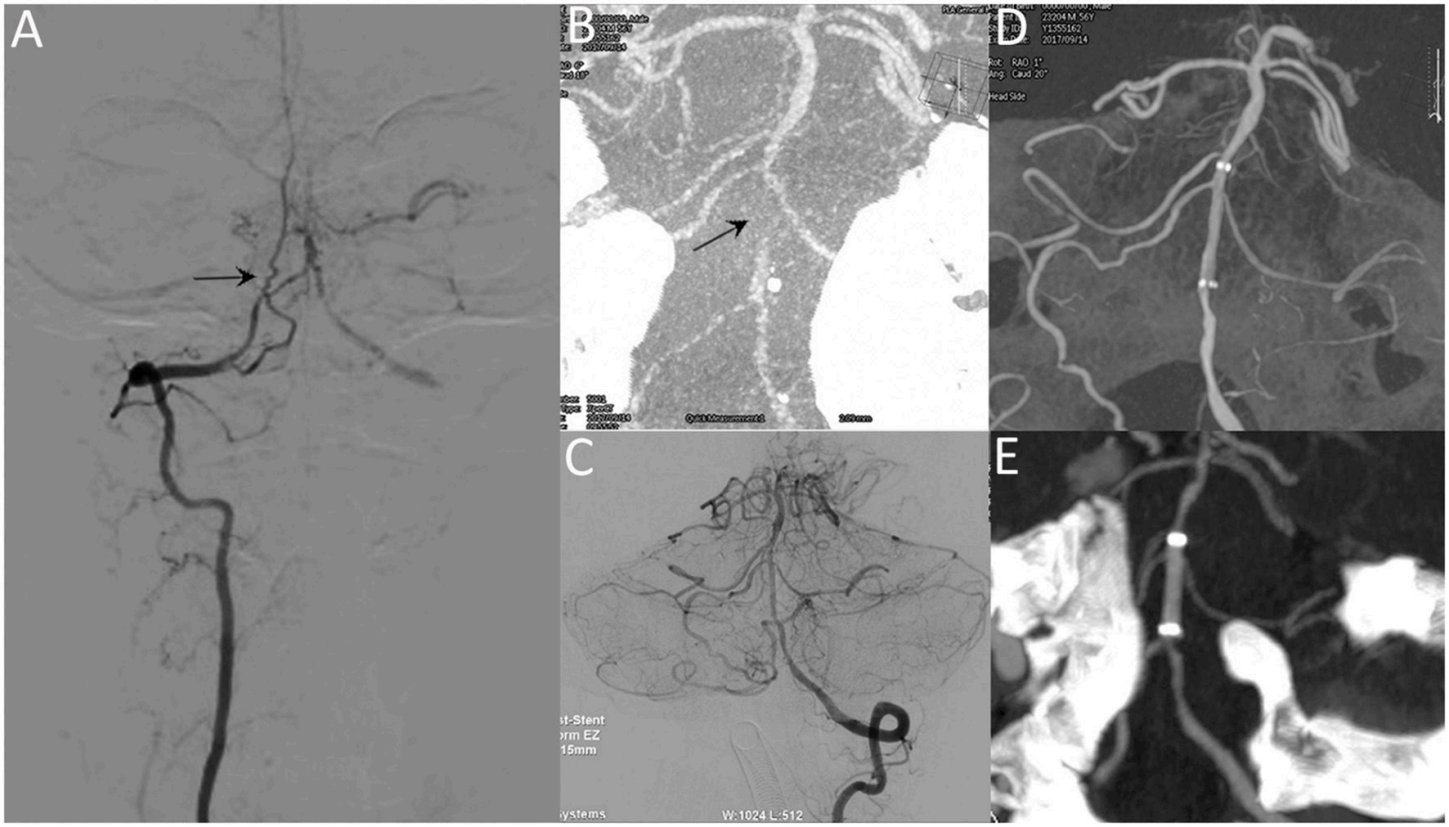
A 53-year-old male with repeated episodes of posterior circulation transient ischemic attacks. Preprocedural DSA image (A) and VasoCT (B) reveal near-occlusion of the basilar artery (arrow). Postprocedural DSA images (C) reveal patency of the basilar artery. Postprocedural XperCT (D) demonstrates good wall apposition of the stent. Follow-up CTA image (E) reveals the patent stent with good apposition 6 months after the procedure.
